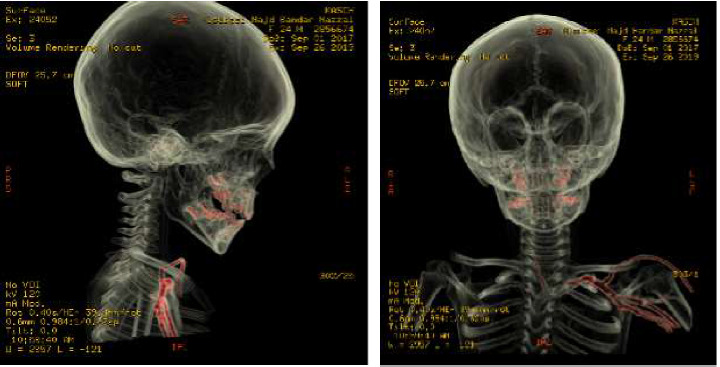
3D from CT angiography with coronal and sagittal views showing bilateral vertebral arteries are normally opacified with redemonstration of intraspinal needle extending from C7-T 1 up to C5-C6. The needle is abutting the right vertebral artery and the anterior spinal artery at the levels of C5 and C6 with no evidence of vascular injury.
